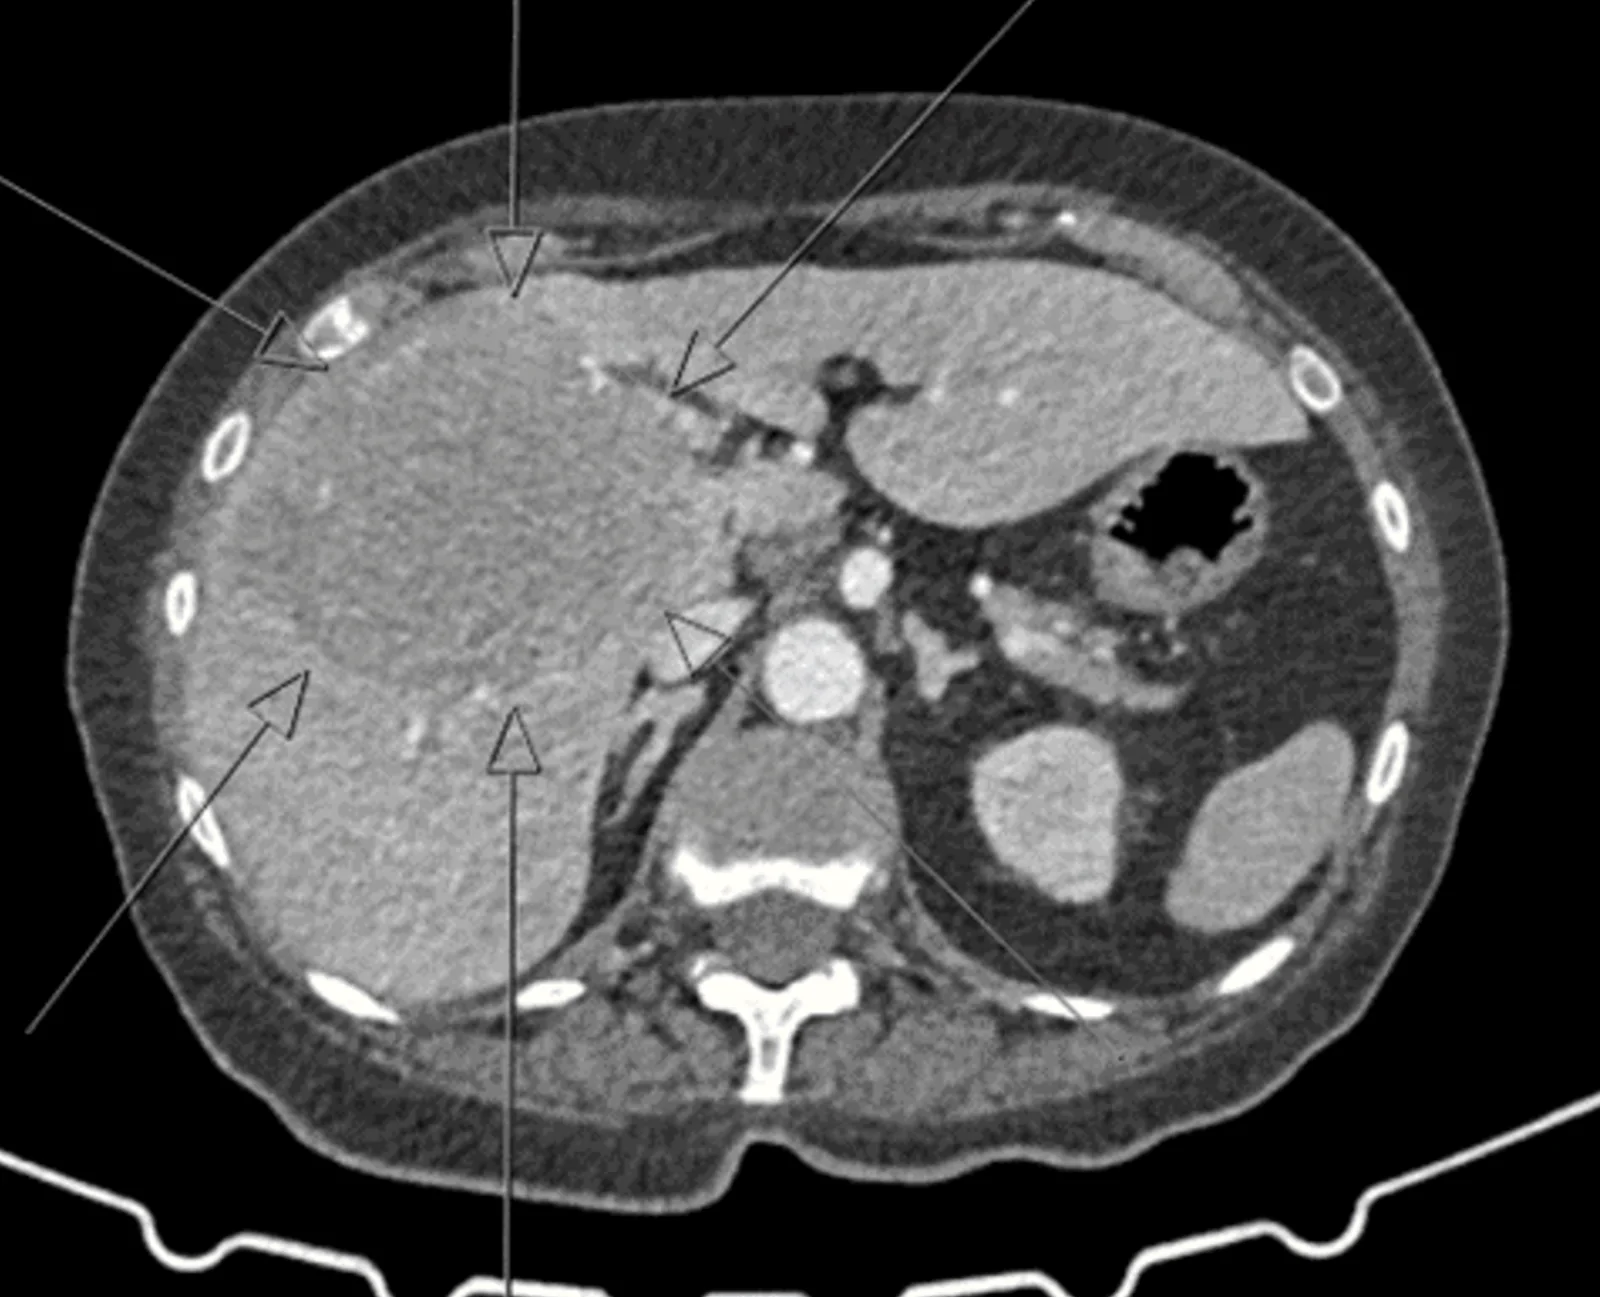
Arterial-phase CT section demonstrating the large mass within the liver Arrows indicate a hypodense hepatic mass lesion
